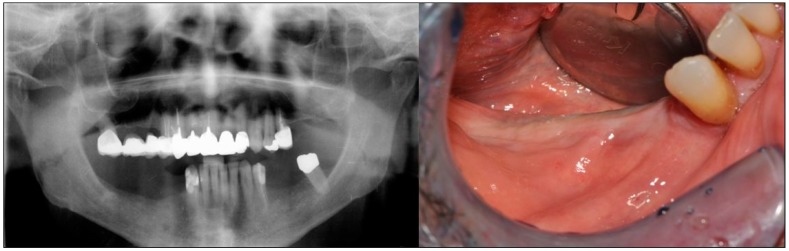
Pre-operative panoramic radiography and intraoral examination. The patient was missing from teeth 4.4 to 4.8.
